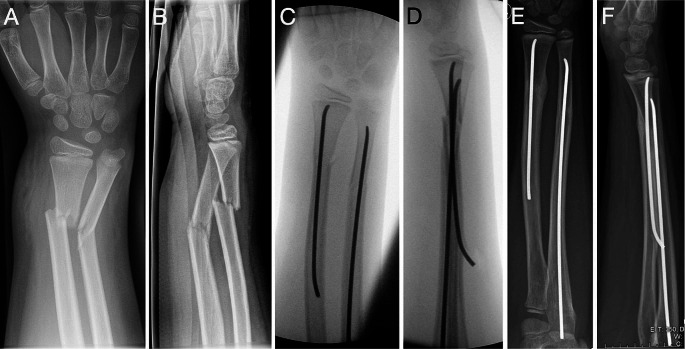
Antegrade ESIN in a 10-year-old boy. Antegrade ESIN in a 10-year-old boy. X-ray at admission (A, B), during surgery (C, D) and before metal removal after 5 months (E, F)
